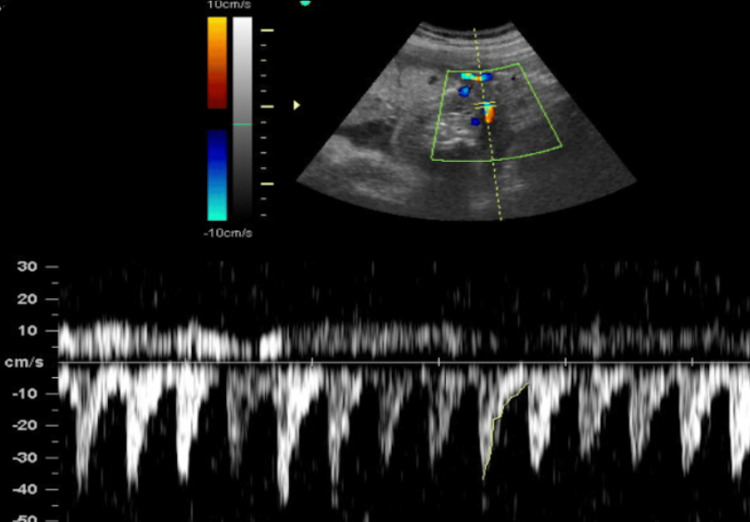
Two-dimensional umbilical Doppler. Above the center line (positive): color flow mapping; below the center line (negative): pulsed-wave Doppler.
